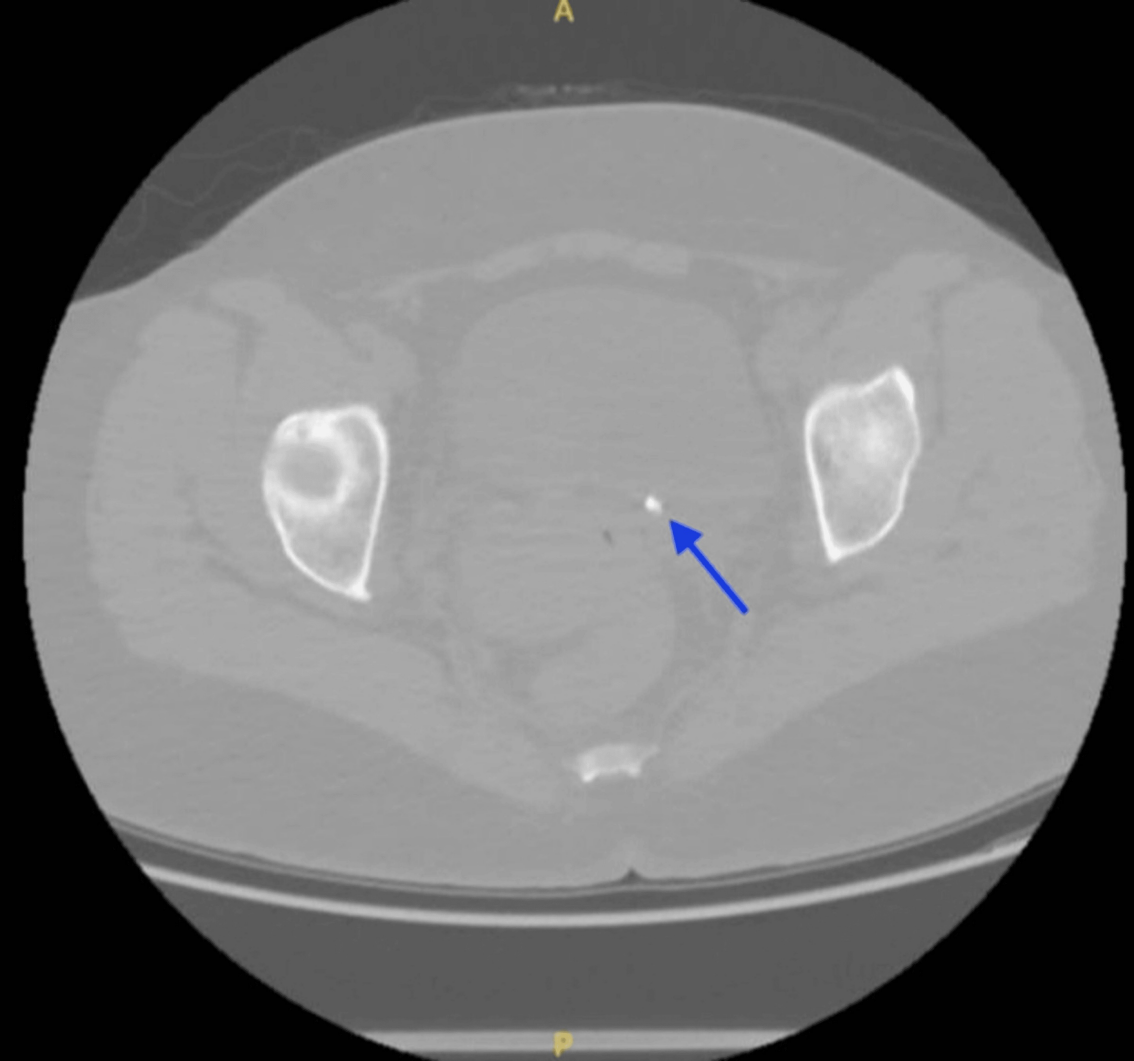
Second Encounter CT-KUB (Axial View) Showing a 6mm left Vesicoureteral Junction Stone (Blue Arrow). CT-KUB: Computed Tomography of the Kidneys, Ureters, and Bladder
